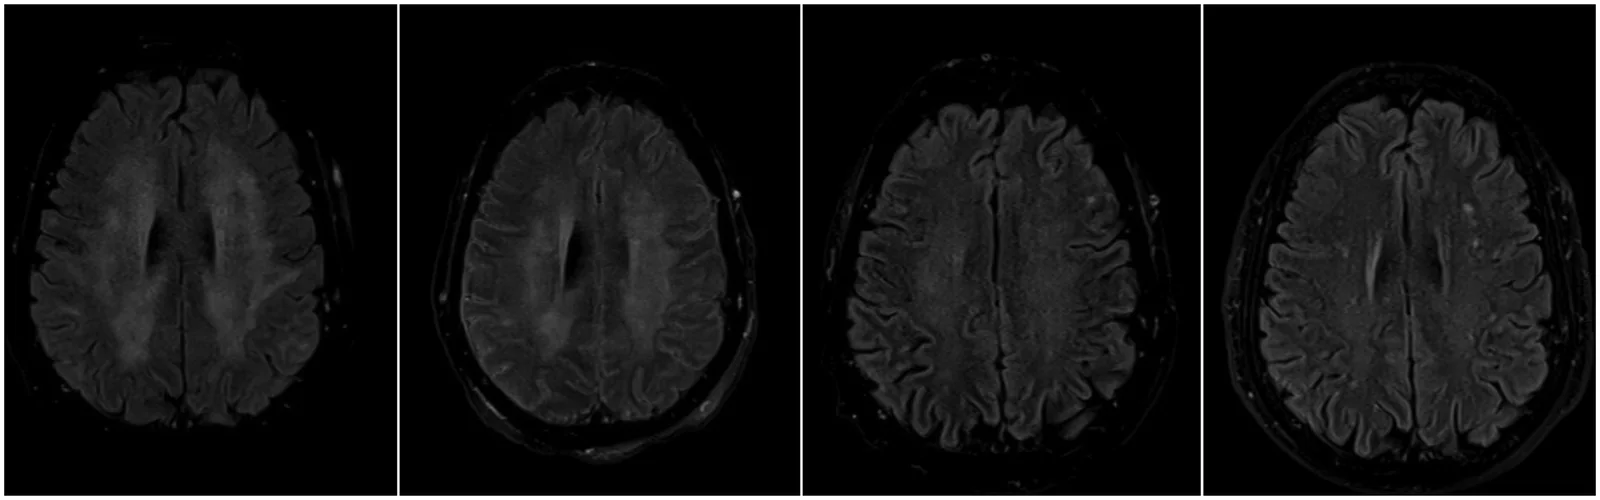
MRI T2/FLAIR sequence showing the progressive subcortical white matter leukoencephalopathy (leftmost image is 10 days post-CAR-T, right most image is pre-CAR-T).
